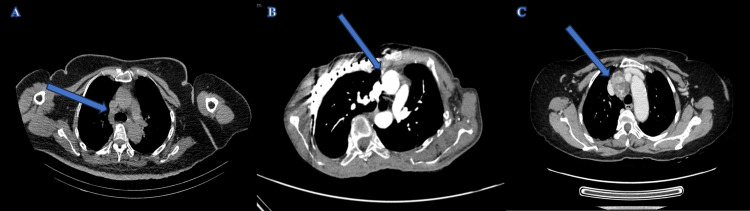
Computed tomography (CT) thorax of Case 1 (A) showed a hypodense lesion (arrow) at the 2R-4R region. Contrasted CT thorax of Case 2 (B) showed a hyperdense lesion (arrow) anterior to ascending aorta. Contrasted CT thorax of Case 3 (C) showed a heterogenous lesion (arrow) located between the right brachiocephalic vein and innominate artery.
